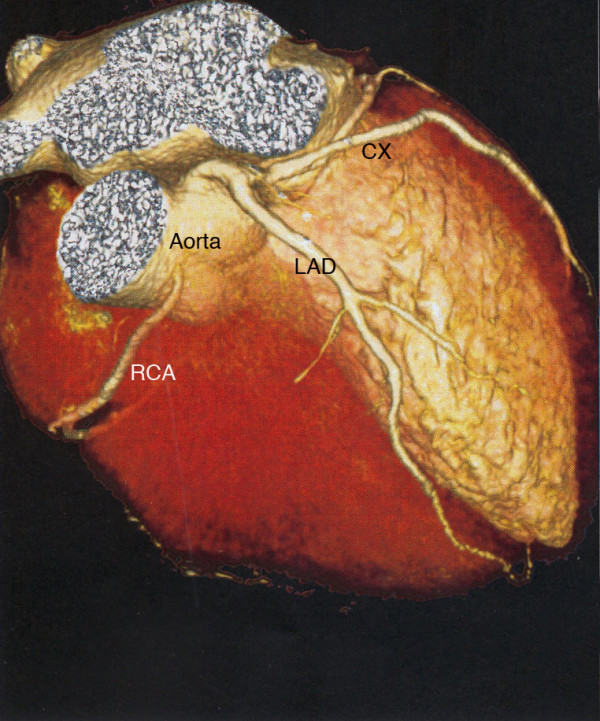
Multislice computed tomography of the heart demonstrates the coronary artery anomaly with a left-side origin of the right coronary artery. CX, circumflex coronary; LAD, left anterior descending artery; RCA, right coronary artery. The right ventricle has been digitally removed.
